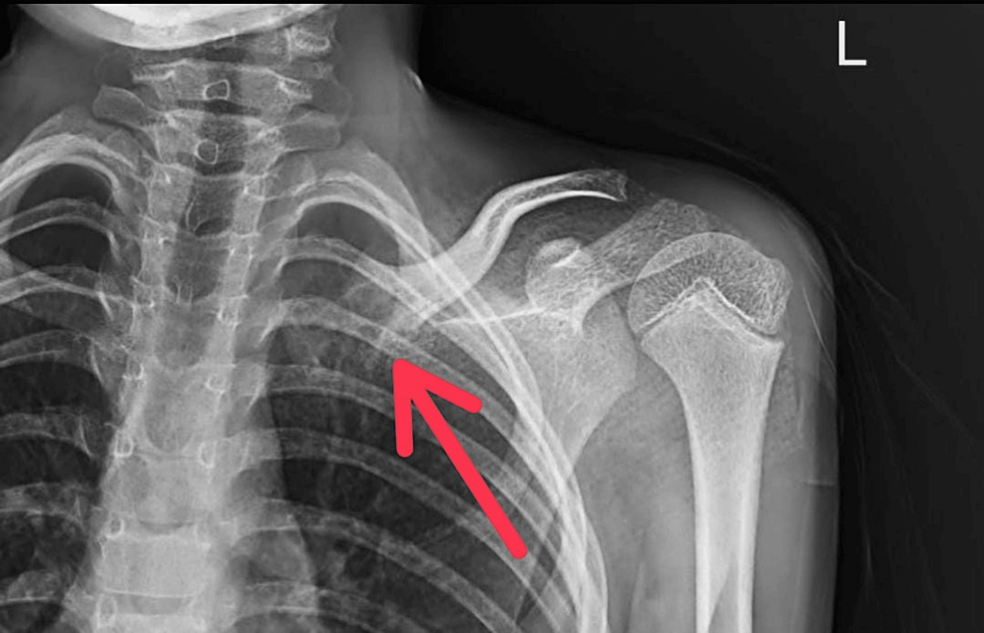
Post-operative X-ray after curettage of the lesion
